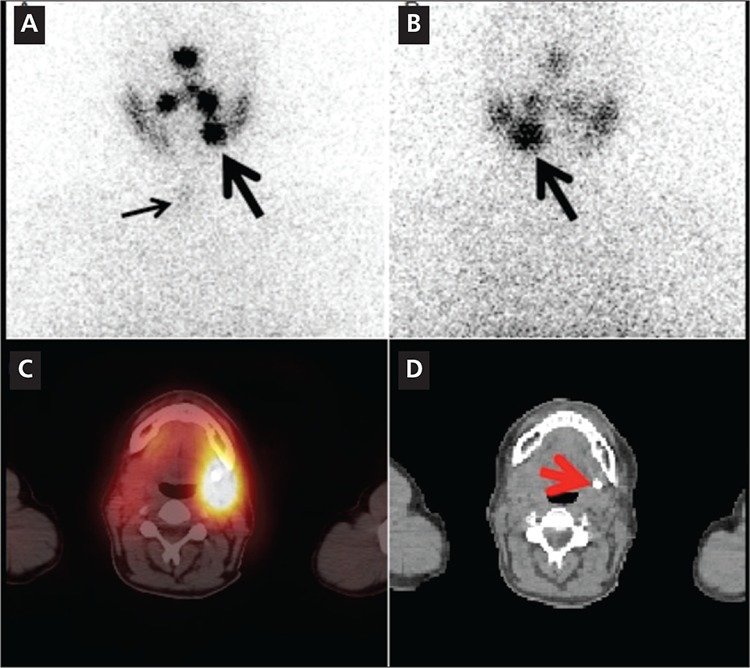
A 42 year-old woman with papillary thyroid cancer and totalthyroidectomy underwent radioiodine therapy with 6031 MBq I-131. 1week post-therapy anterior (A) and posterior (B) neck/chest images showedmild uptake in the thyroid bed (small arrow, image A) consistent withminimal residual thyroid tissue. A focus of intense increased asymmetricuptake noted in the left inferior head/neck region was concerning for nodalmetastasis (large arrows, images A, B). Axial fused SPECT/CT and axial CTimages (C, D). Focus of asymmetric increased uptake localizes to thesubmandibular gland. CT images show an 8 mm calcified stone immediatelyinferior to the left submandibular gland, causing duct obstruction andasymmetric uptake in the salivary gland (red arrow, D). On SPECT alone thiswas suspected to be a metastasis, but the addition of CT provided accuratecharacterization as a benign finding
